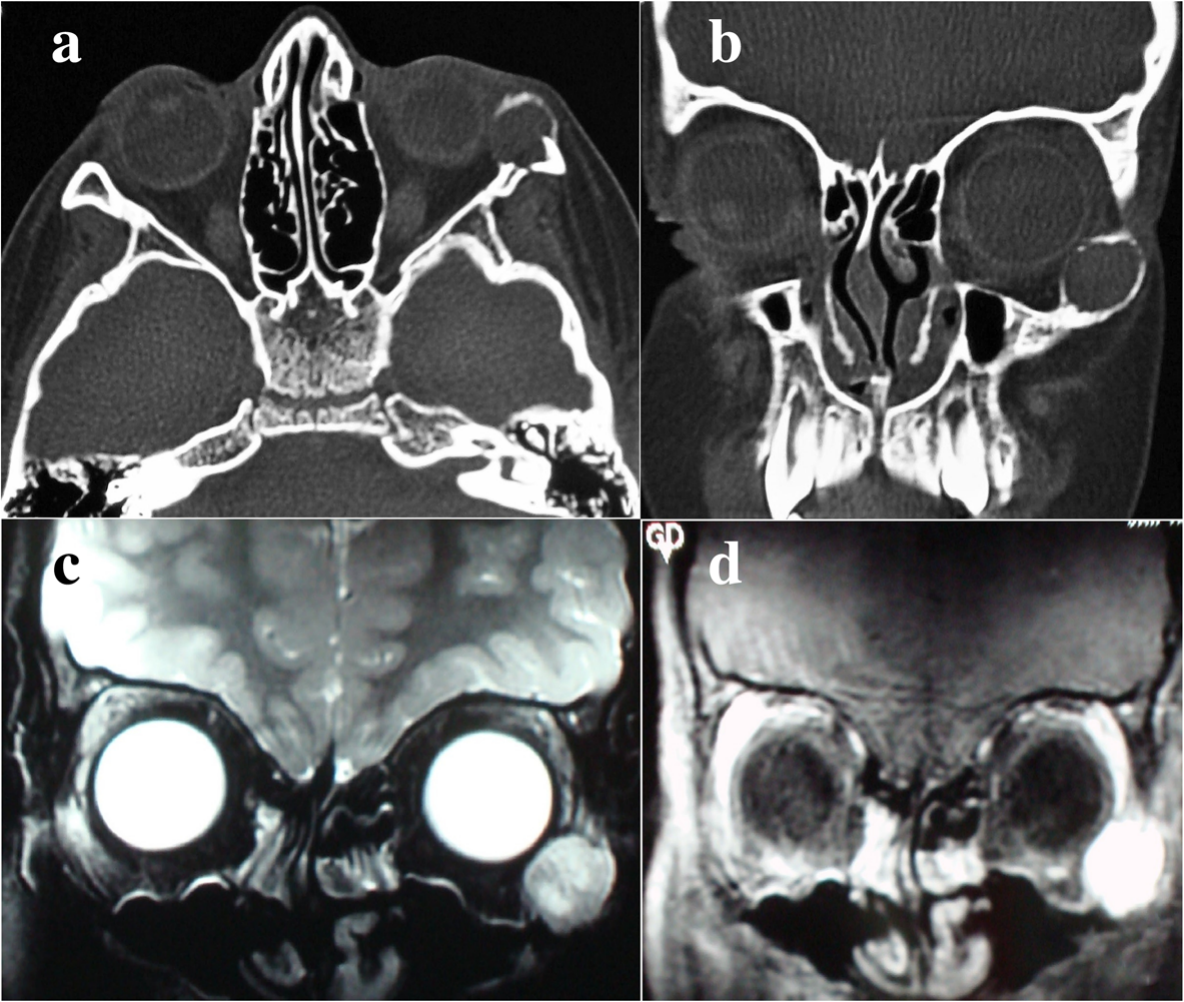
Preoperative imaging. CT scan reveals an isodense intraosseous lesion in the zygomatic bone in axial (a) and coronal sections (b). In orbital MRI, the mass appears heterogenous in T2-weighted images (c) and shows remarkable contrast enhancement after gadolinium injection (d)
